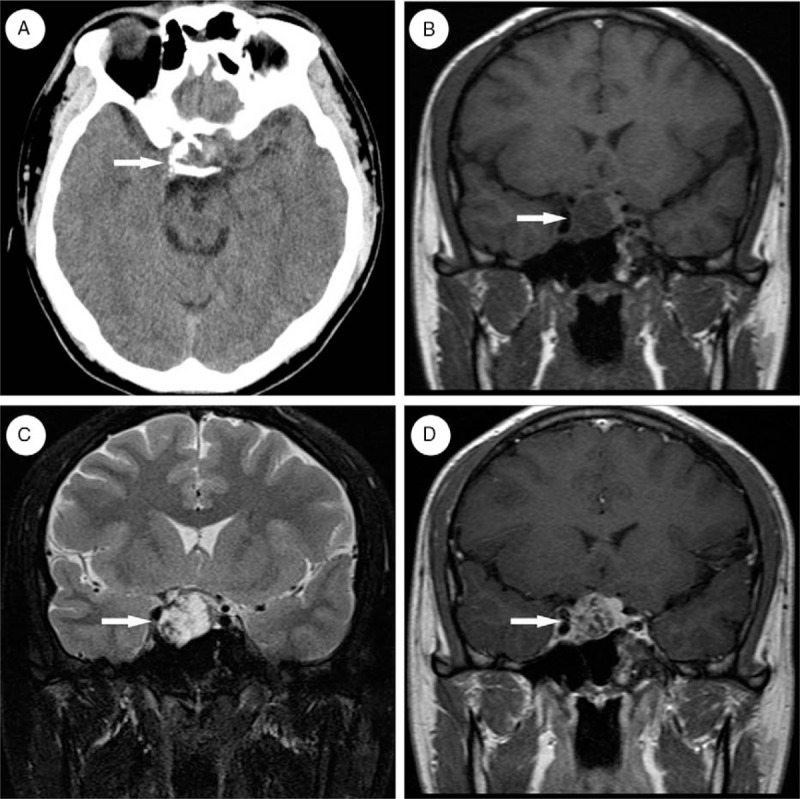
(A) Computed tomography (CT) scan showed intermediate-to-high density shadow of clusters in sellar region with multiple calcification nodules at the boundary, lesion was marked with white arrow. (B) Hypointensity T1-weighted image, lesion was marked with white arrow. (C) Hyperintensity T2-weighted image, lesion was marked with white arrow. (D) Inhomogeneous contrast enhancement of T1-weighted image, lesion was marked with white arrow.
